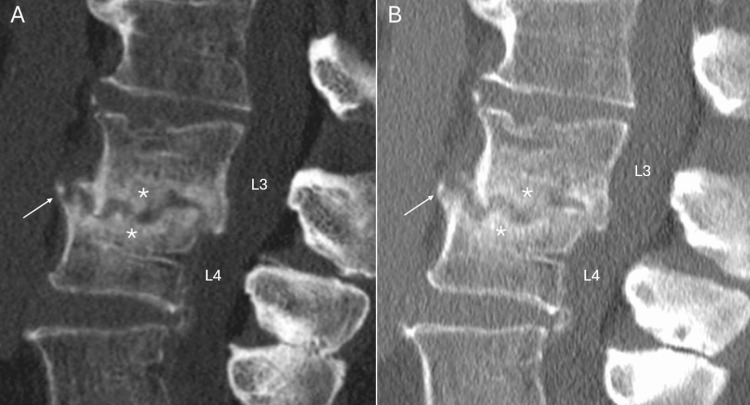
Sagittal reconstruction of lumbar spine CT scan, taken two months (A) and seven months (B) after the end of treatment. Follow-up CT scan showed bone reformation, seen as sclerosis of trabecular bone (stars) and osteophytosis (arrows), with no signs of progressive erosions, consistent with post-infectious ankylosis of L3-L4. CT, computed tomography
